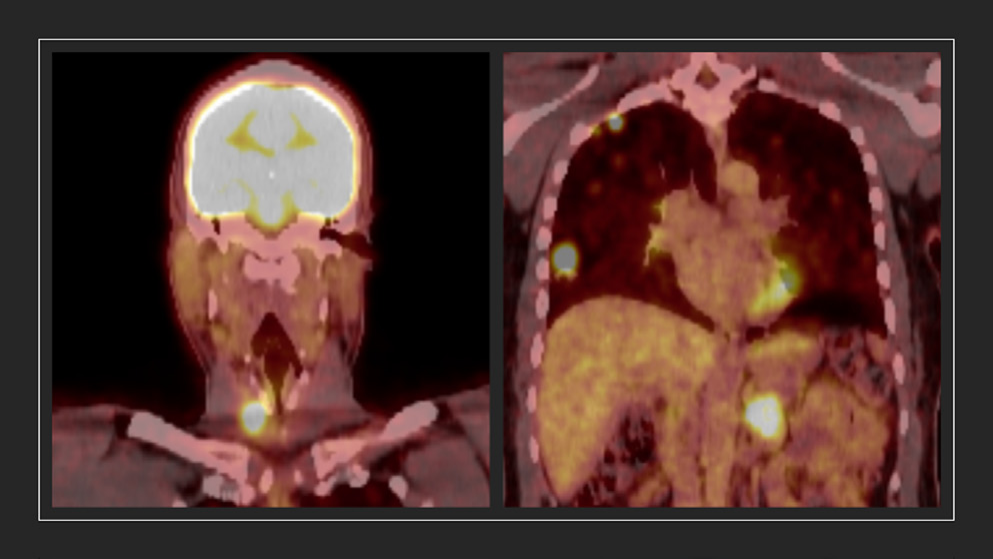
FDG-PET scan revealed a 3.0 × 1.7 cm (transverse by AP dimensions) right thyroid bed mass with an SUV_max_ of greater than 10, multiple metastatic pulmonary nodules, including a 2.0 × 1.8 cm in the medial right middle lobe, and a 3.2 × 3.4 cm left retroperitoneal mass with an SUV_max_ of 8.3.
